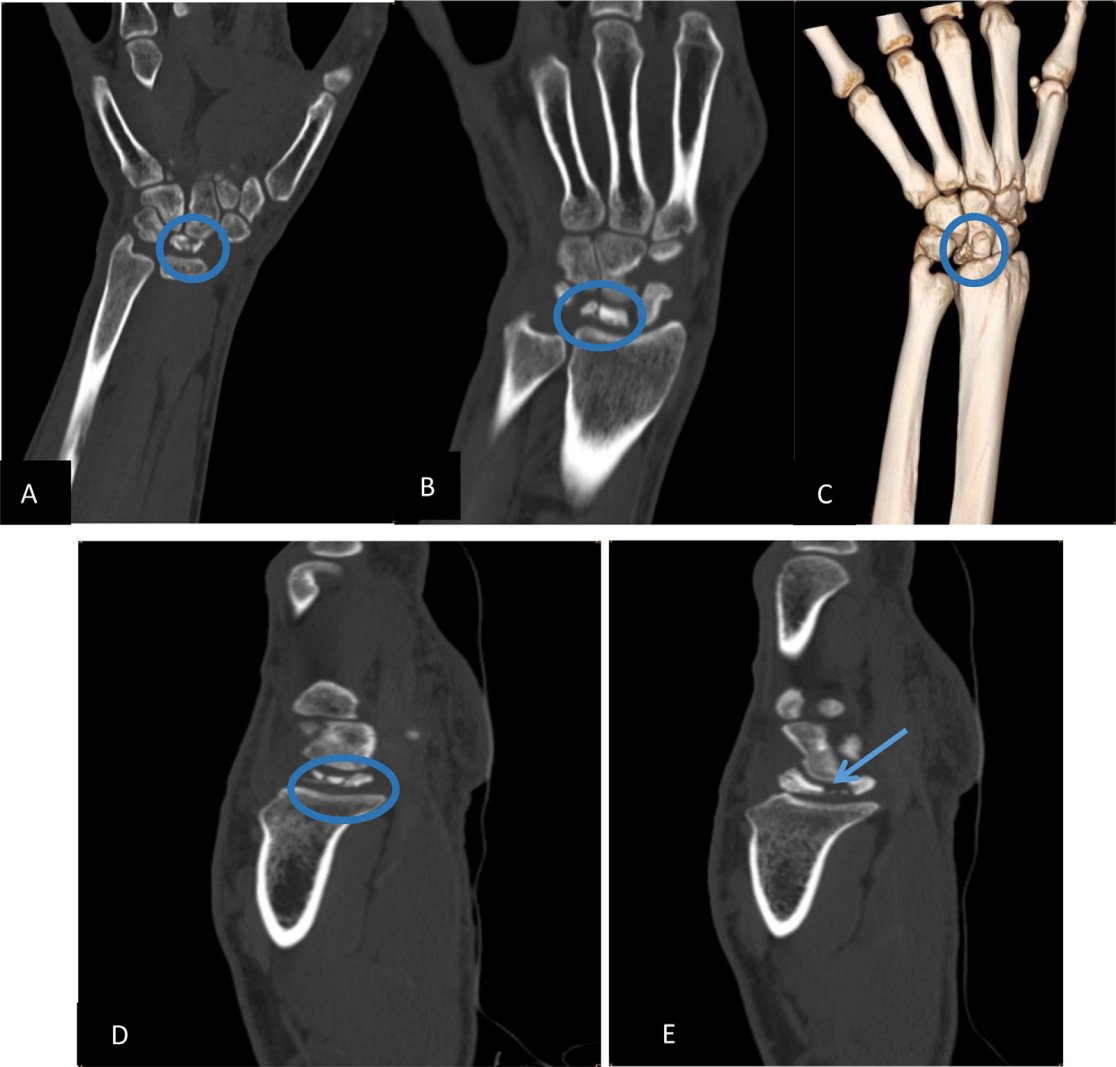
CT of the left wrist in coronal (A and B), sagittal (D and E), and 3D (C) sections revealing sclerosis and fragmentation of the lunate with articular erosion.
